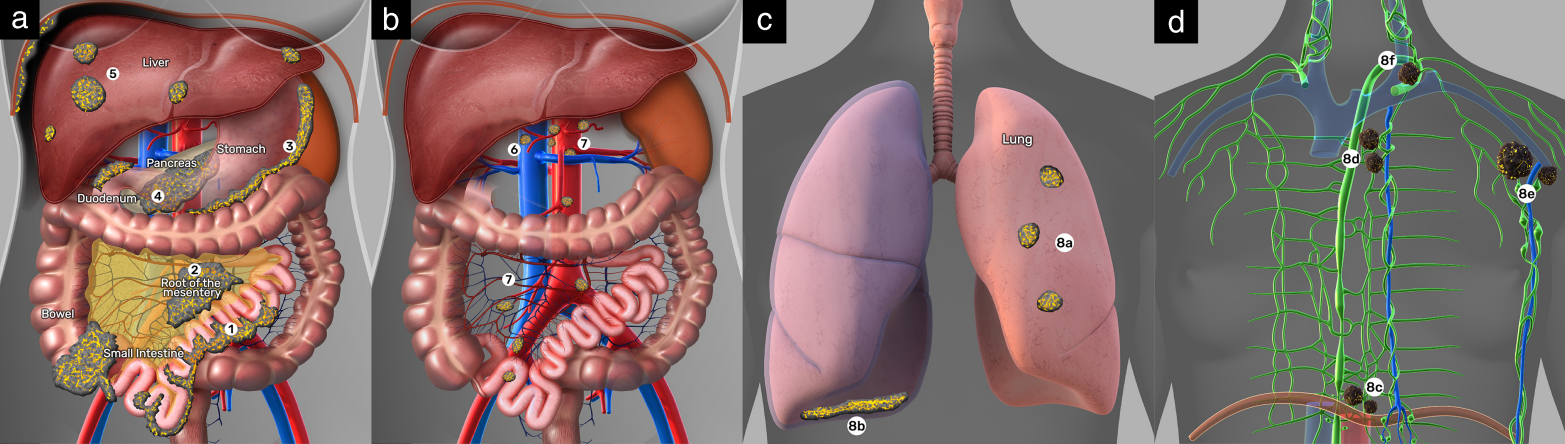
Anatomical sites for the European Society for Medical Oncology (ESMO) and European Society of Gynaecological Oncology (ESGO) criteria for non‐resectability of tubo‐ovarian carcinoma^19^: (a) small intestine (1), small‐bowel mesentery (2), stomach/duodenum (3), pancreas (4) and liver (5); (b) hepatic hilum and celiac trunk (6) and abdominal lymph nodes (7); and (c,d) extra‐abdominal sites (8). Examples of extra‐abdominal involvement: lung metastases (8a), parietal pleura (8b) and superior diaphragmatic (8c), parasternal (8d), axillary (8e) and supraclavicular (8f) lymph nodes. For detailed description of criteria, see Table 1.
